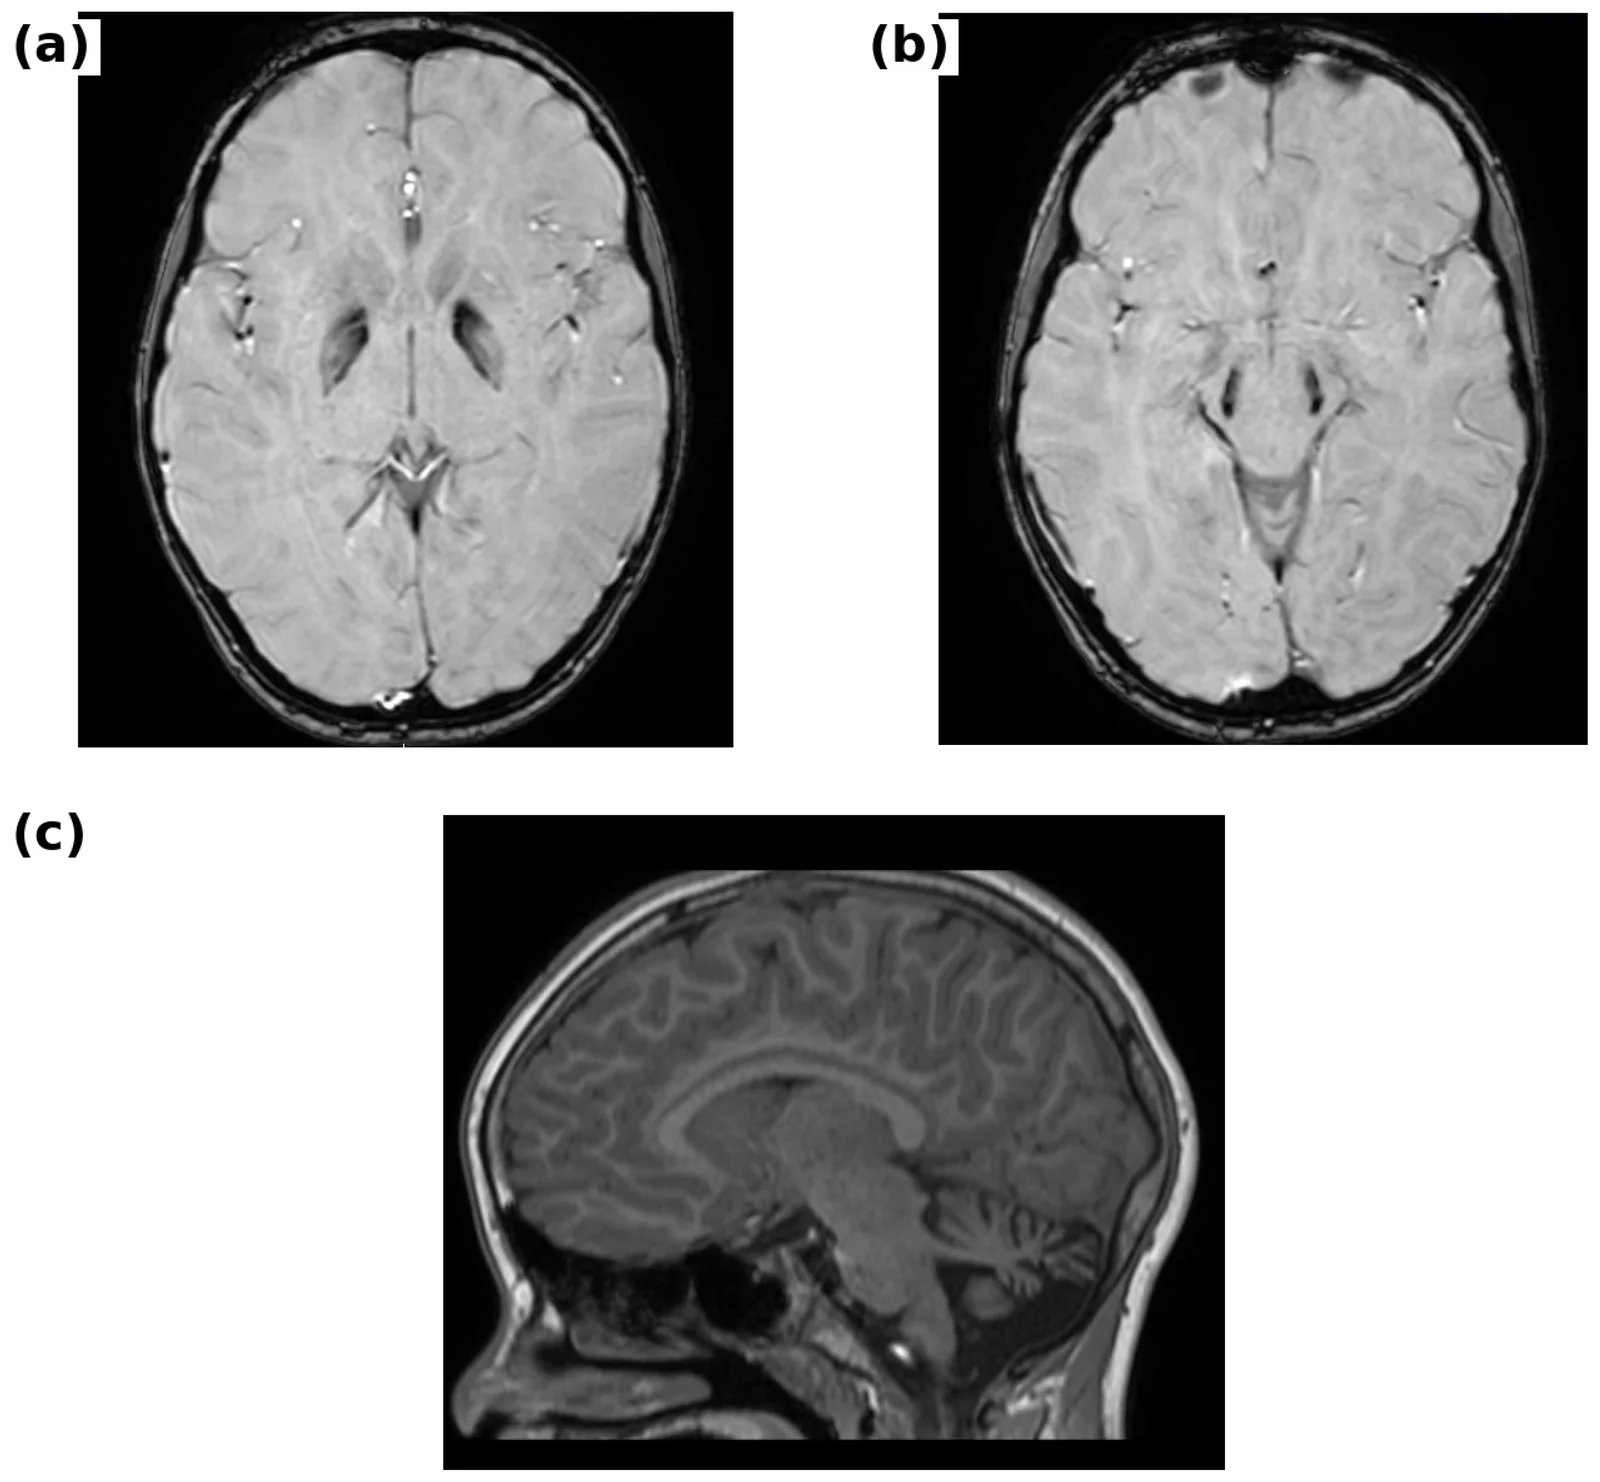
Brain MRI of Patient 2. (a) Axial susceptibility-weighted image showing bilateral hypointensity of the globus pallidus. (b) Axial susceptibility-weighted image showing hypointensity of the substantia nigra. (c) Sagittal T1-weighted image showing marked cerebellar atrophy.
